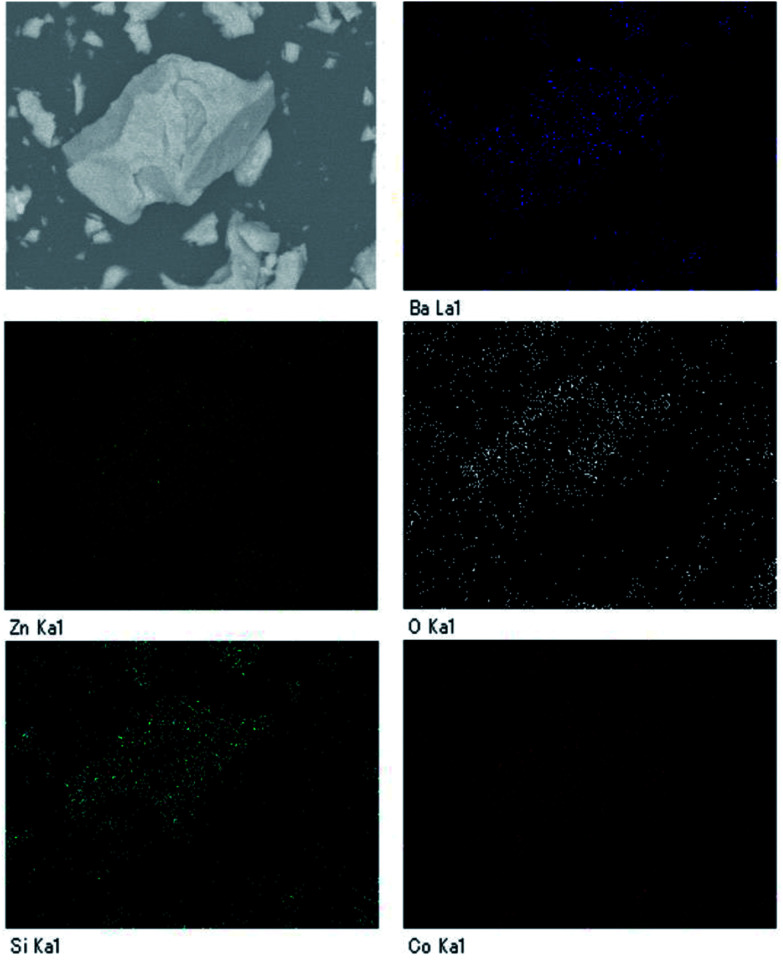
The X-ray dot mapping analysis of the Ba(Zn_0.85_Co_0.15_)_2_Si_2_O_7_.
